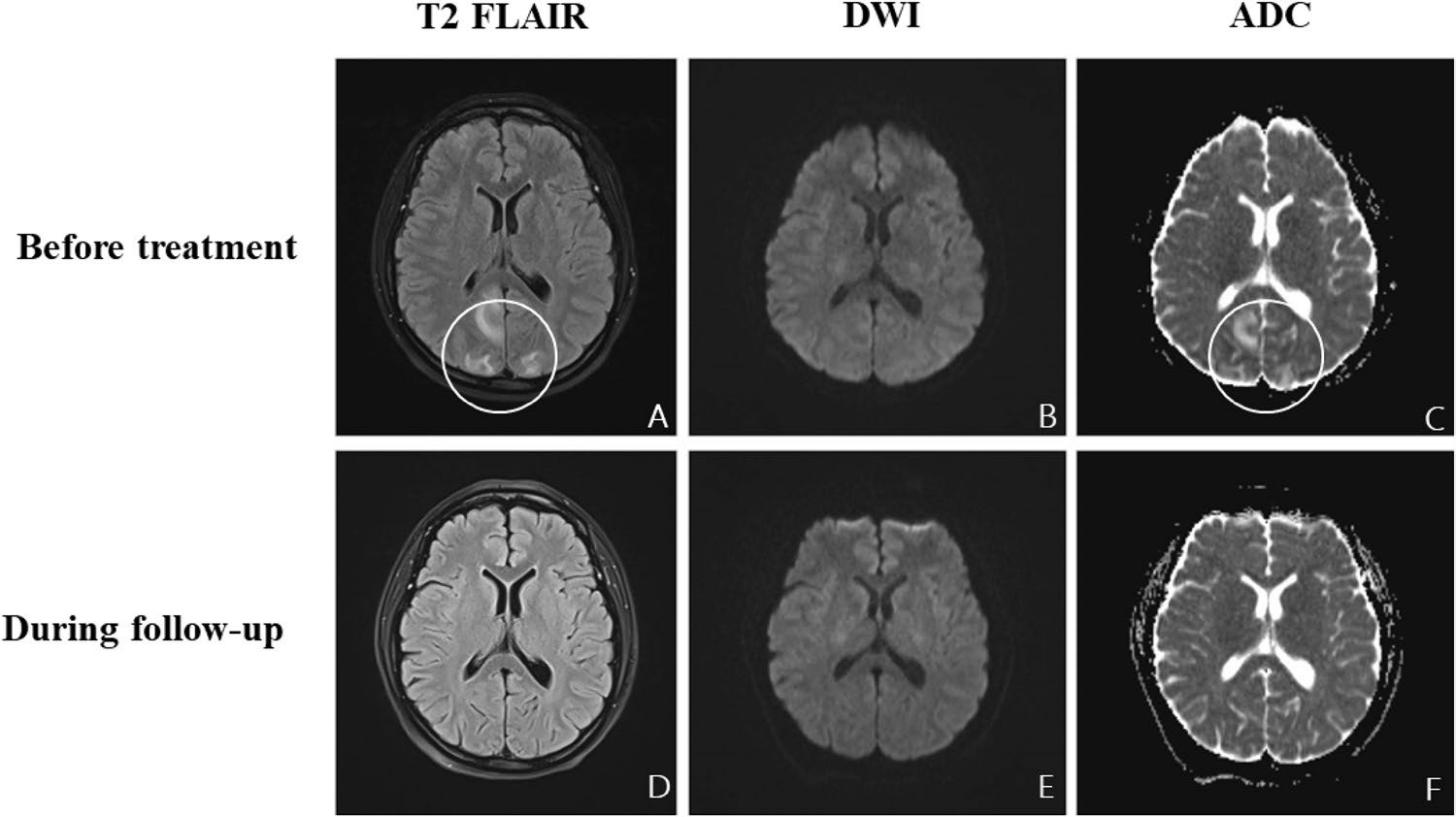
Brain MRI in Patient 6. A–C show brain imaging examined before treatment, and D–F show imaging during the follow-up period. A Hyperintensity present on bilateral parietal occipital lobes on T2-FLAIR and ADC imaging (C). No abnormal intensity was observed on DWI (B). D–F The lesions were fully absorbed, and no abnormal intensity was found on imaging taken during the follow-up period
